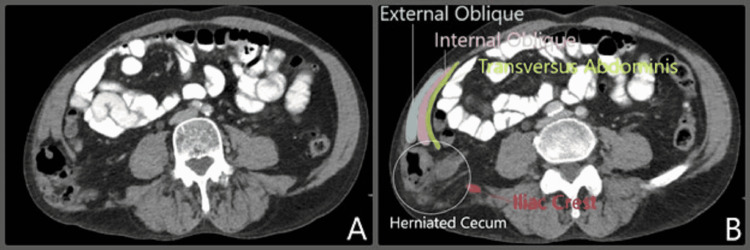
(A) Preoperative CT of the abdomen/pelvis, axial view, demonstrating a right lumbar defect now containing cecum. (B) Same image, structures labeled
